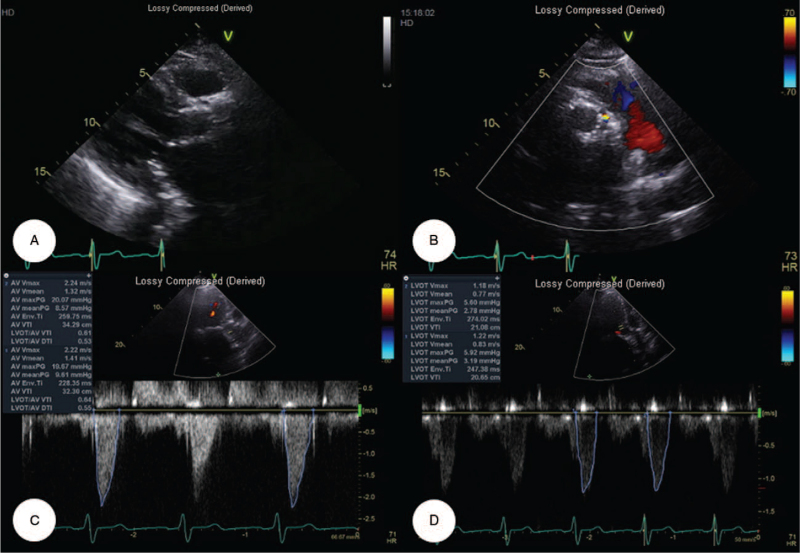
Follow-up transthoracic echocardiography. (A, B) Parasternal long- and short-axis views revealed that the valve prosthesis (CoreValve^TM^ Evolut R^TM^, Medtronic Inc., Minneapolis, MN) was satisfactorily expanded and apposed, with mild paravalvular leakage. (C, D) Peak velocity had declined from the initial 4.54 to 2.22–2.24 m/s, and the mean pressure gradient had also declined from 48.5 to 8.57–9.61 mm Hg.
